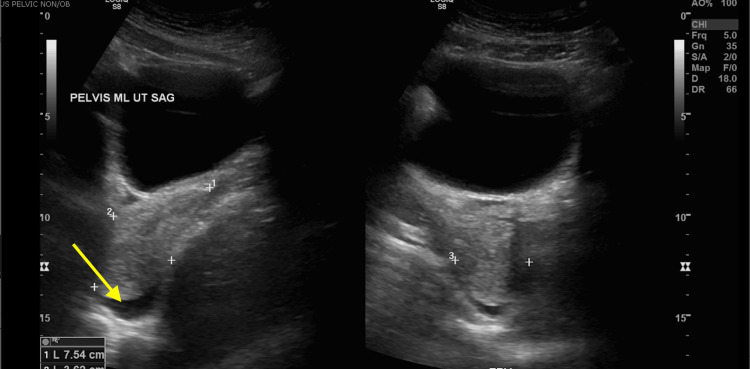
(Left, right) Pelvic ultrasound Impression: Uterus size of 7.5cm craniocaudal. Small volume pelvic free fluid. Otherwise negative study.
